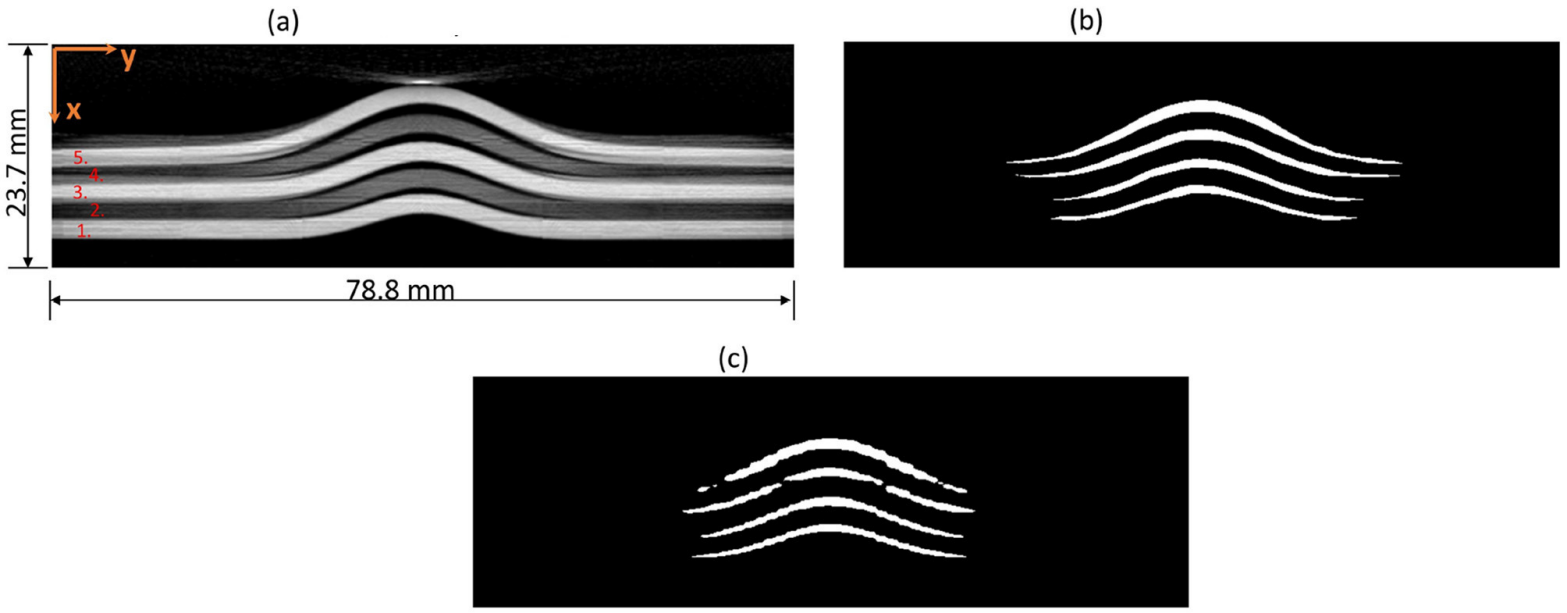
(a) An example CT slice of synthetic data along with the layer numbering shown in red text. (b) The hand-annotated mask. (c) The mask predicted by the detectron2.
